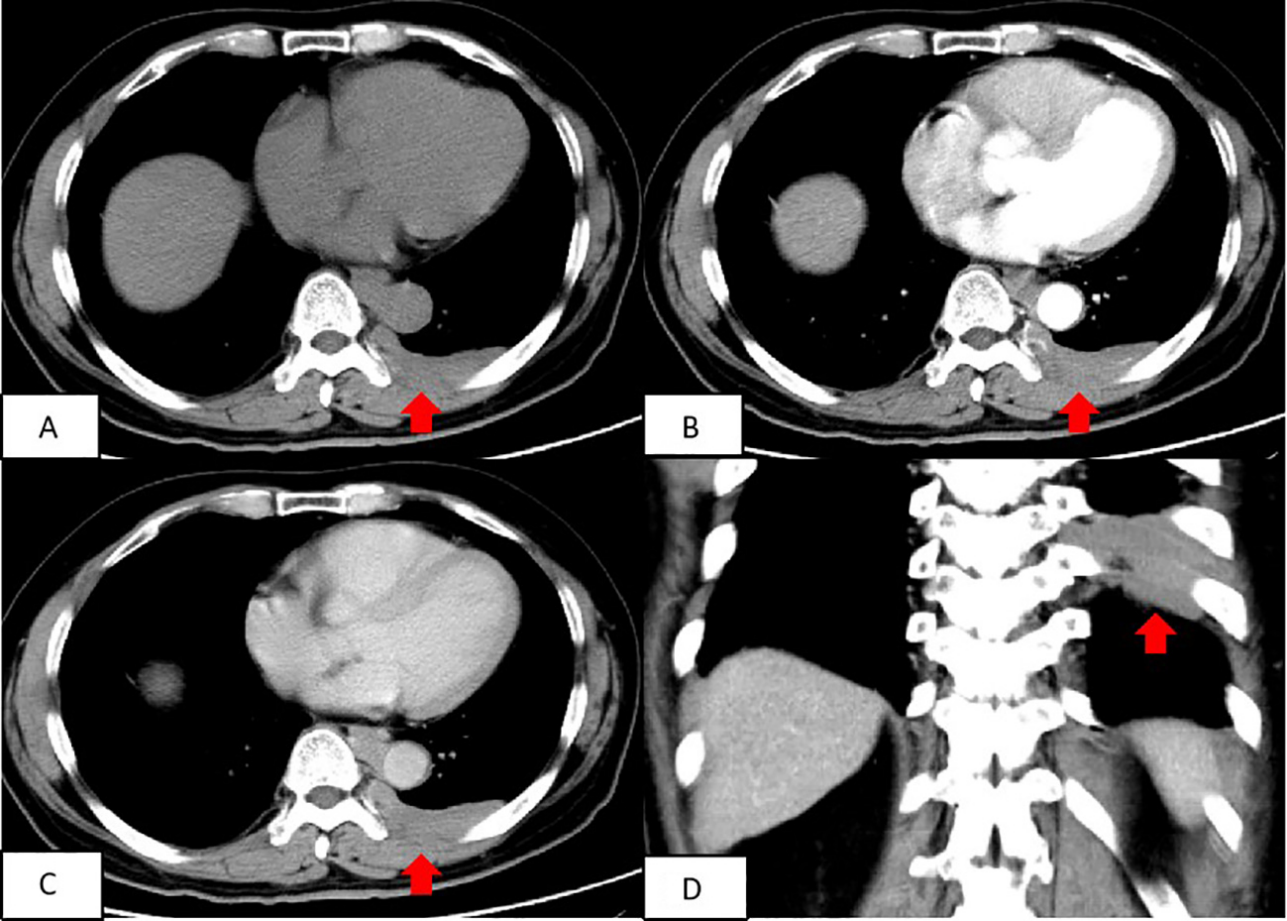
Contrast-enhanced CT scan of the chest depicted a round and flaky soft tissue density shadow in the thoracic aorta and the left lower pleura area, both with uniform density. The size of the lesion was approximately 6.8roximat The shadow was closely associated with the pleura and exhibited mild enhancement on the enhanced scan, with small blood vessel shadows passing through it. (A) Transverse position CT plain scan; (B) Transection CT scan arterial phase; (C) Transection CT scan venous phase; (D) Coronal position CT scan.
